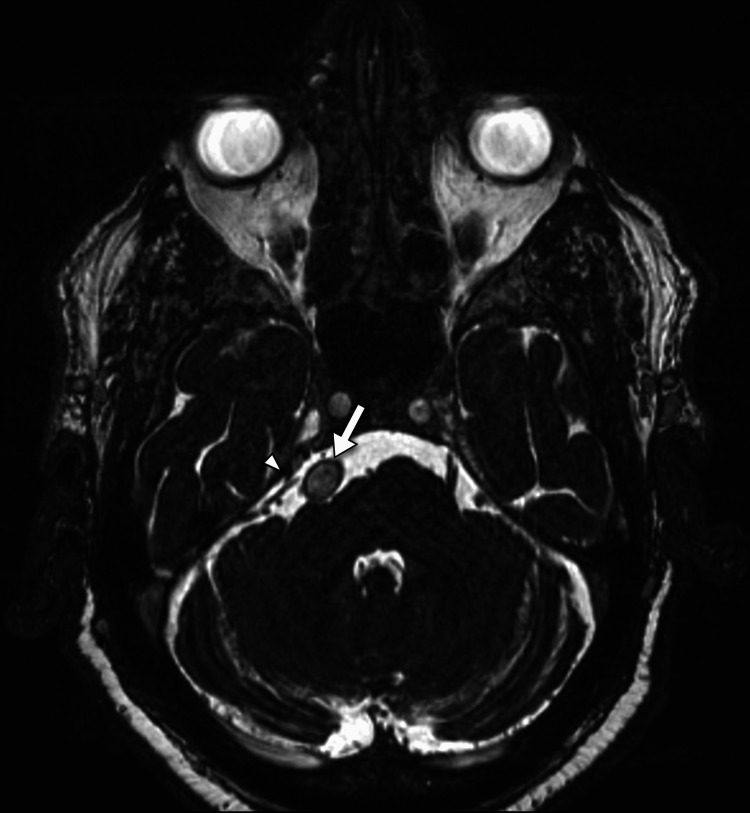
An axial image of the patient's brain MRI in the CISS sequence shows the dilated basilar artery (arrow) compressing and displacing the right trigeminal nerve (arrowhead).
